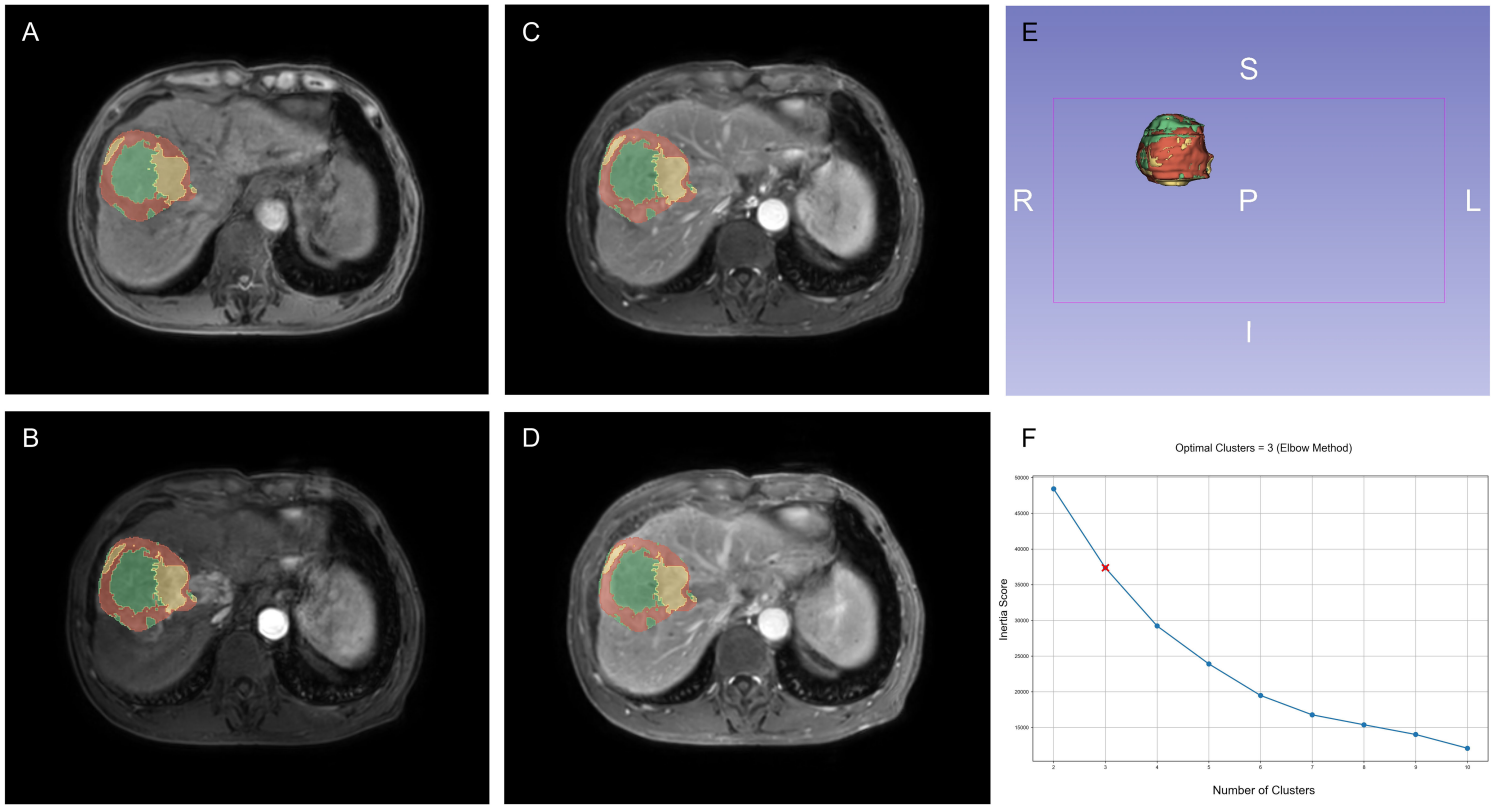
Habitat subregion segmentation across multiphase MRI and clustering validation. (A) Pre-contrast. (B) Arterial phase. (C) Portal venous phase. (D) Delayed phase. (E) 3D view of the ROI divided into three habitat subregions. (F) Determination of the optimal number of clusters using the Elbow method.
